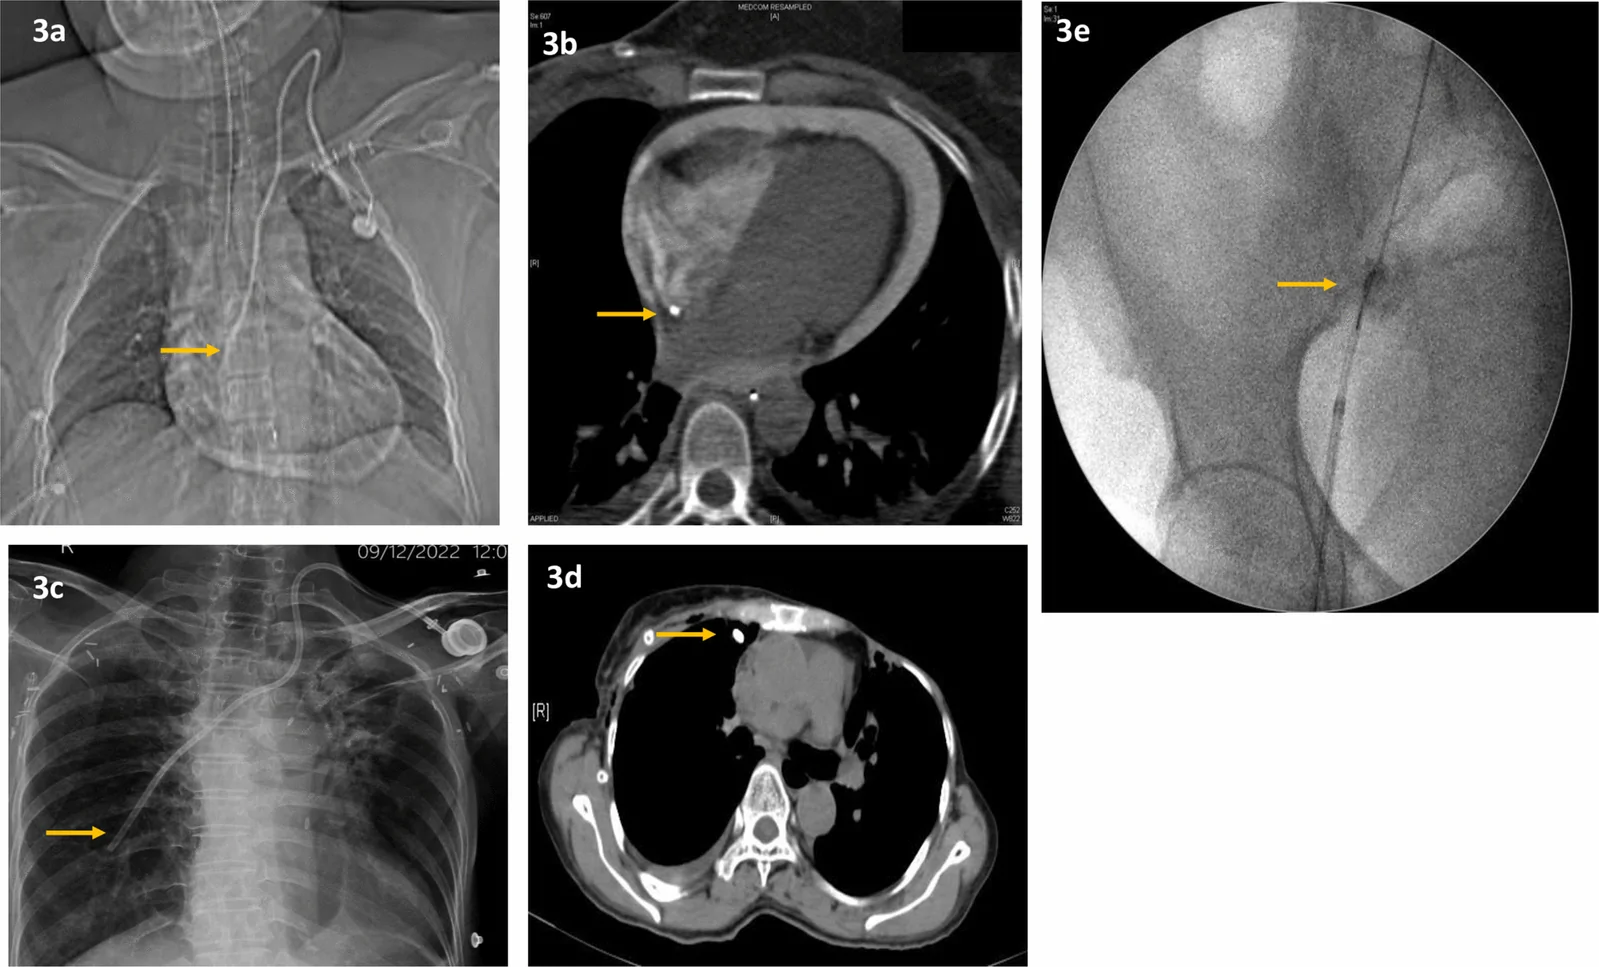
Major Complications. 3a- Portogram suggestive of PORT tip in the pericardium, 3b- CT portogram showing dye in the pericardial cavity, 3c- Chest X-ray suggestive of pleural placement, 3d- CT thorax sagittal view confirming PORT tip in the pleural cavity, 3e- Fluoroscopic image showing snare retrieval of guide wire from the external iliac vein
